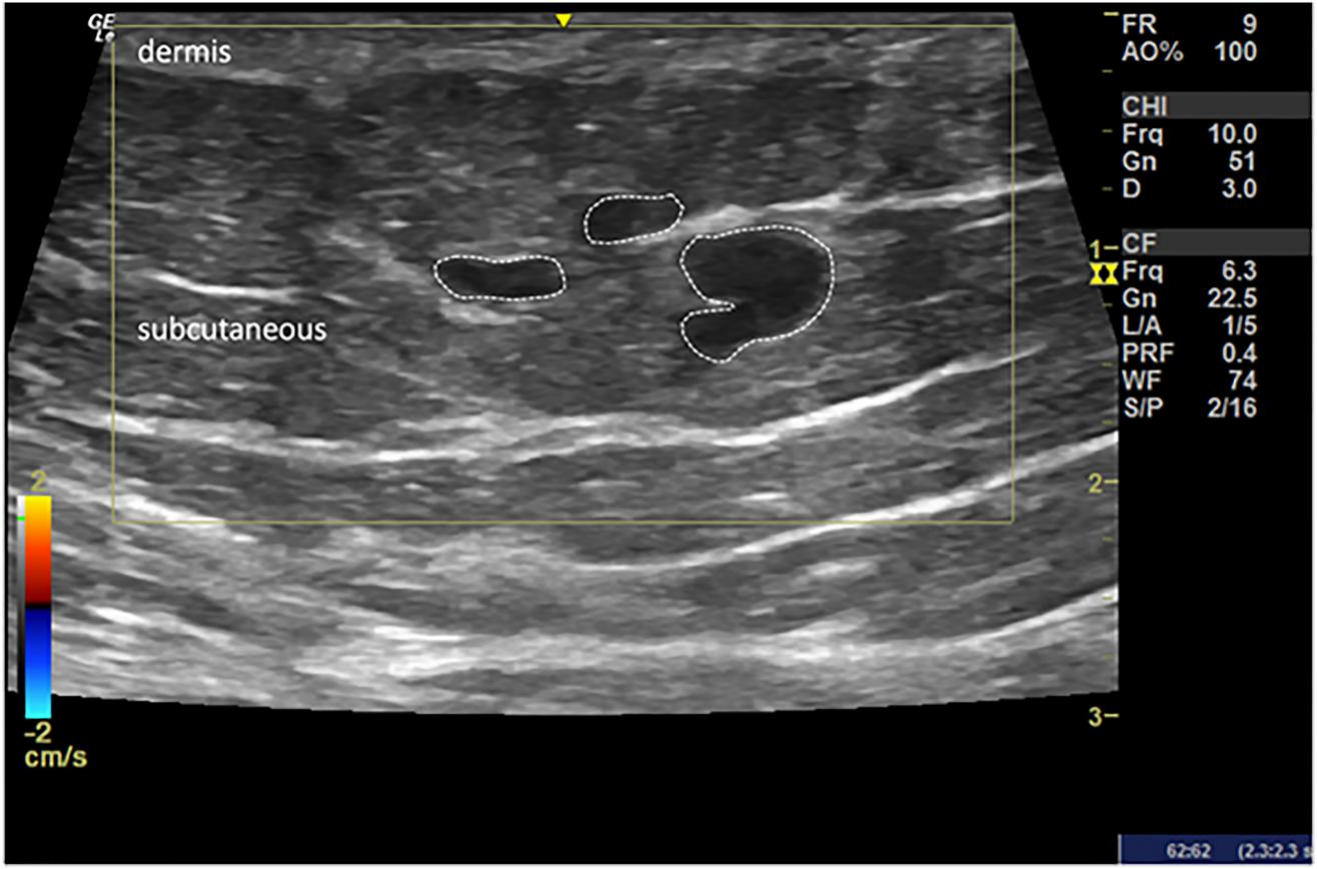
Colour Doppler ultrasound image of the plan chosen. Dotted lines represent the deposits of hyaluronic acid (HA) placed on a plan where no major vessels were found.
